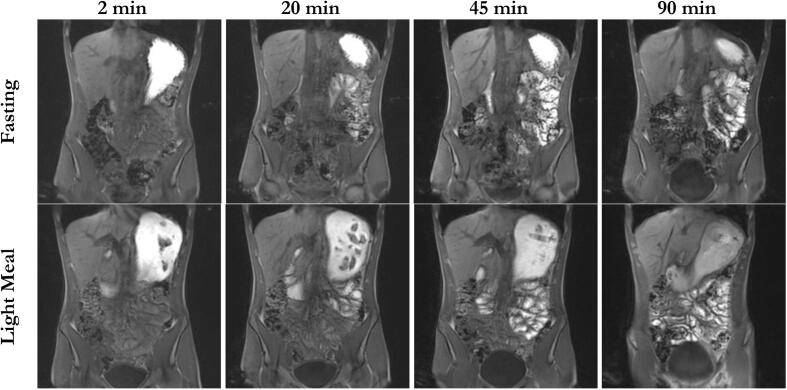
Coronal T1-weighted VIBE MR images of participant 8. For each study arm, images were selected to display the most distal contrasted areas in the gastrointestinal tract. Images were taken at 2, 20, 45 and 90 min after ingestion of 240 mL of a manganese labeled glucose solution in the fasted state and after ingestion of a light meal. White areas indicate that the lumen/ tissue is in contact with the manganese solution.
